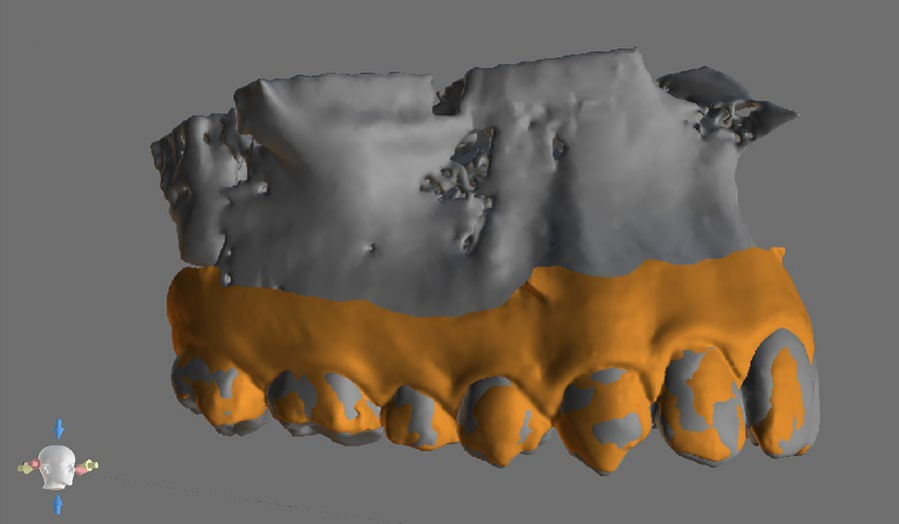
CBCT scan and maxillary digital model superimposition
